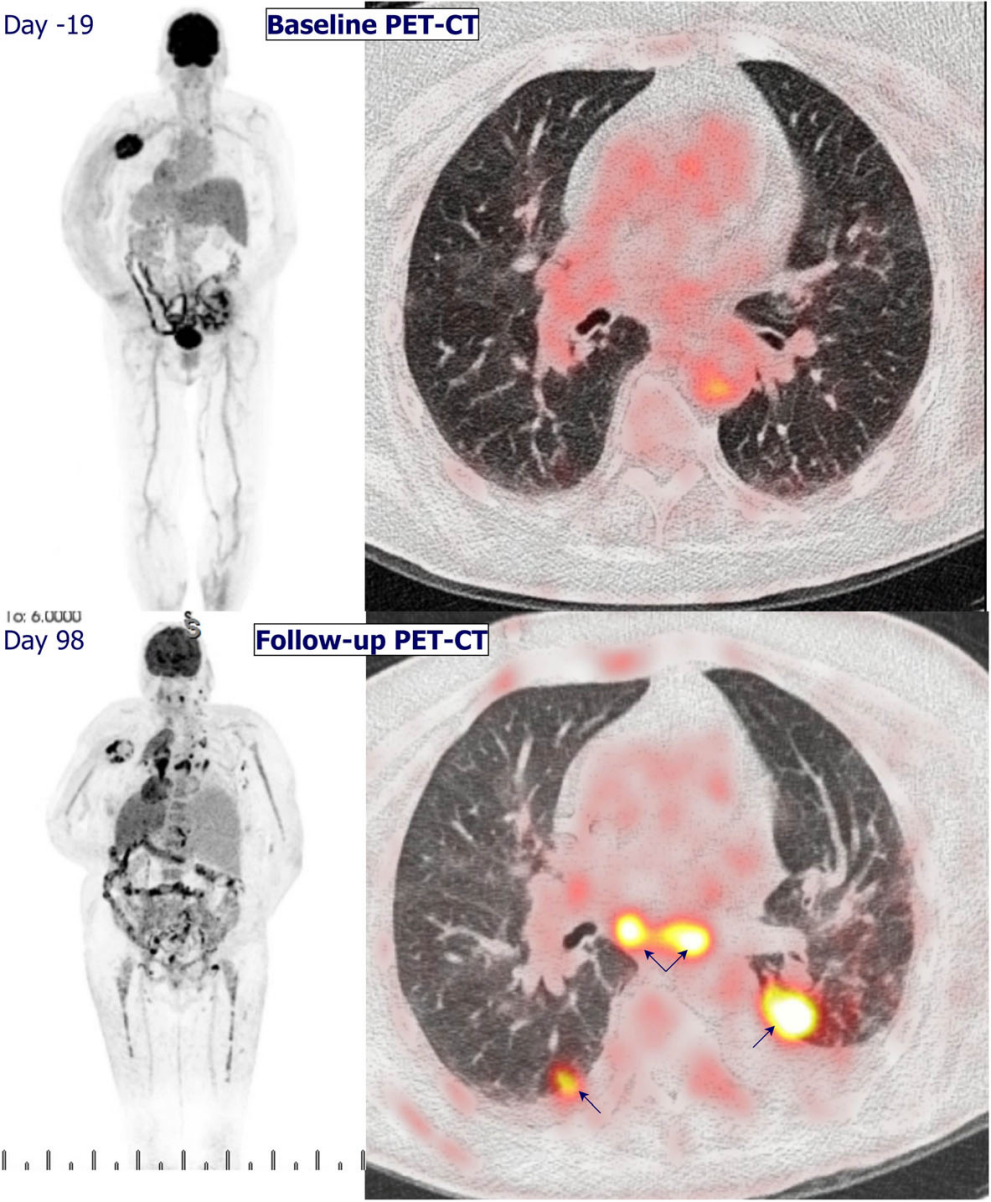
^18^F-FDG PET-CT revealed progressive disease at three months after start of nivolumab, with new lung and lymph node metastases. Pleural effusion was present
